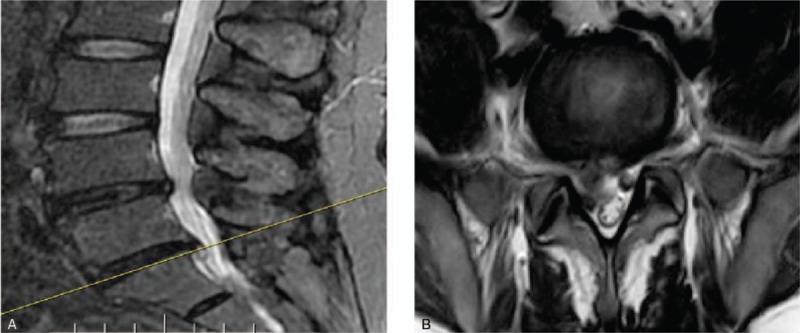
Pre-operative MRI of lumbar disc herniation at L4 L5 in sagital view and axial view. (A) Pre-operative MRI of lumbar disc herniation at L4 L5 in sagital view. (B) Pre-operative MRI of lumbar disc herniation at L4 with compression of nerve root of the left foramen (axial view).
